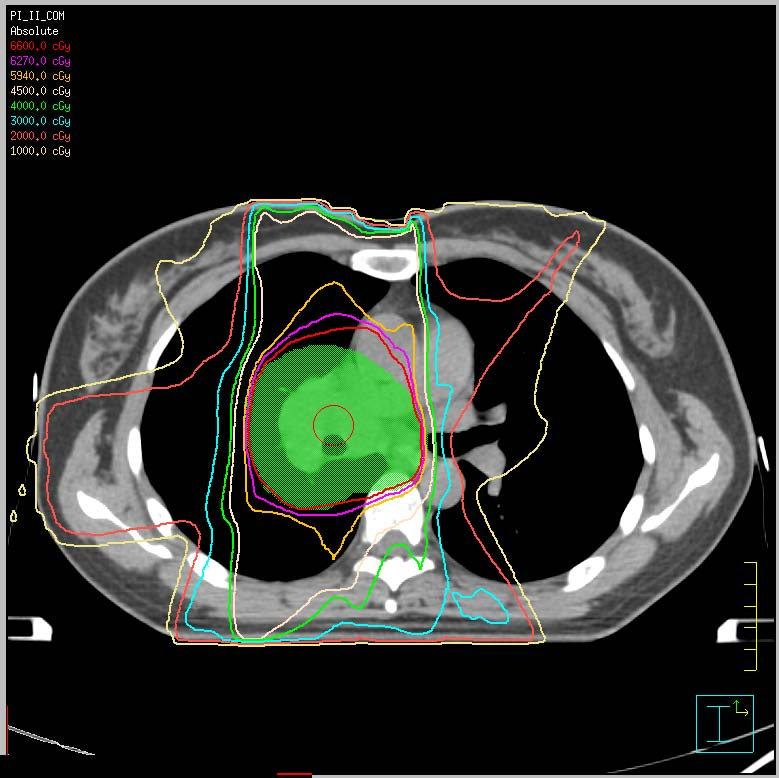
CT based IMRT plan of Patient 10.
